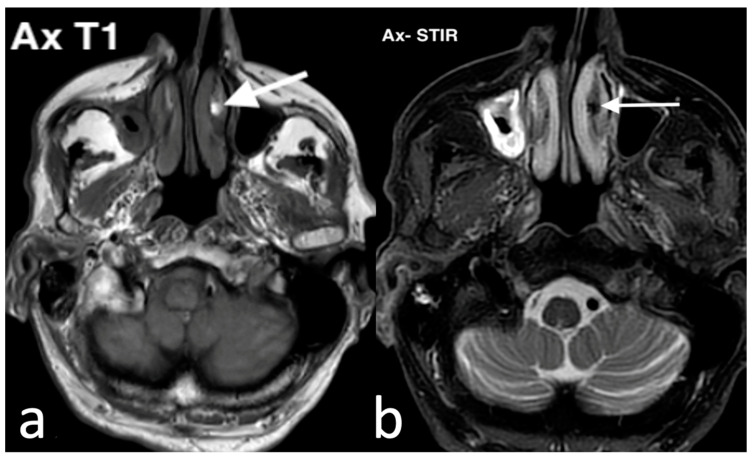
Lipoma in the inferior left nasal turbinate as an incidental finding on MRI. On axial T1 image (a), a millimetric oval spot in the inferior left nasal turbinate with high intensity (arrow) is observed; on axial STIR image (b), lipoma typically shows signal drop (arrow) due to fat signal suppression.
